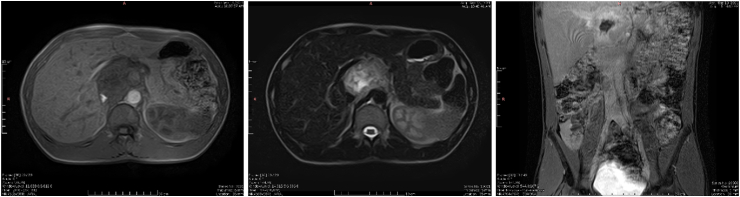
Axial and coronal planes demonstrating an iso-to hypo-intense mass on unenhanced T1-weighted images (left), and iso-to hyper-intense on fat-saturated T2-weighted images (center), with avid and heterogenous enhancement (hyperintense rim with hypointense central region) on gadolinium-enhanced T1-weighted images (right).
